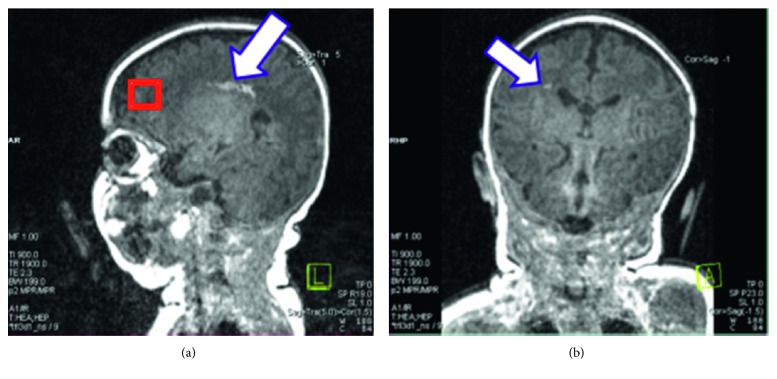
Infant's MRI results reveal periventricular leukomalacia in both the left (a) and right (b) sides of brain.
